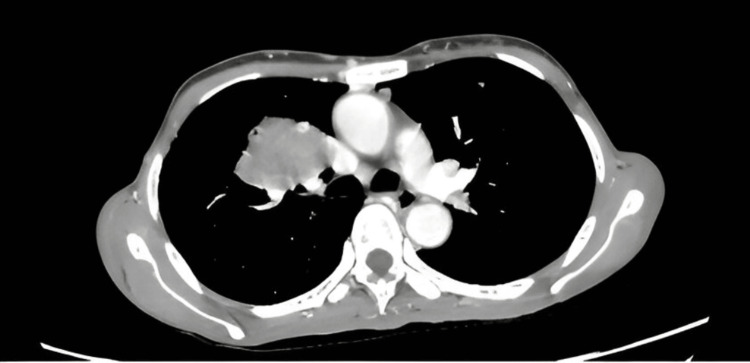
CT scan shows multiple rounded lesions with spiculated margins in bilateral lungs. CT, computed tomography
